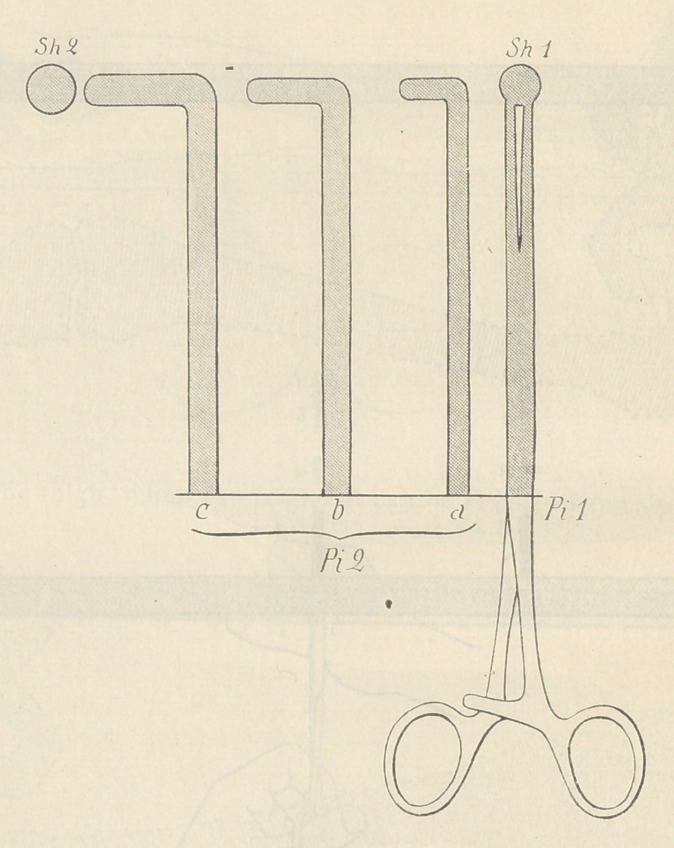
# Method of Localization and Extraction of Projectiles by the Simultaneous Use of Two X-Ray Tubes
